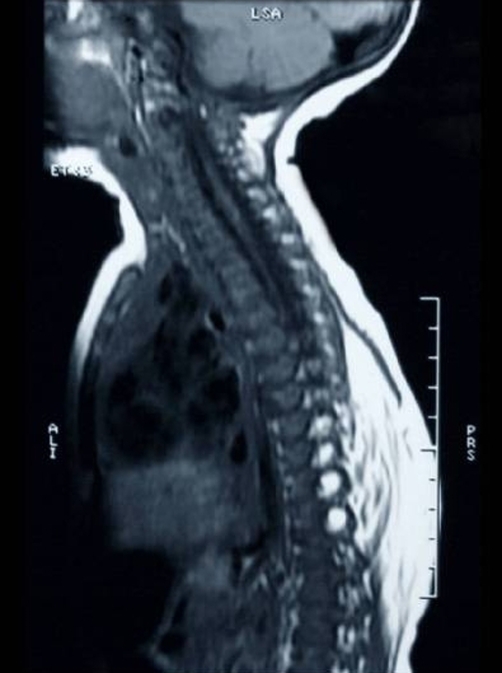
Sagital MRI demonstrating involvement of the lower thoracic region by LMMC.
